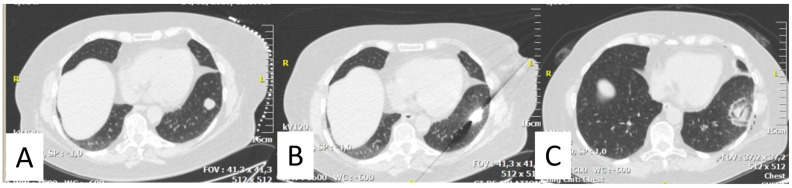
(A) 59 years-old female sarcoma patient with a solitary metastasis of the left lung; (B) CT-guided microwave ablation was performed; (C) Metastasis was completely ablated with safety margins (ground glass infiltrate).
